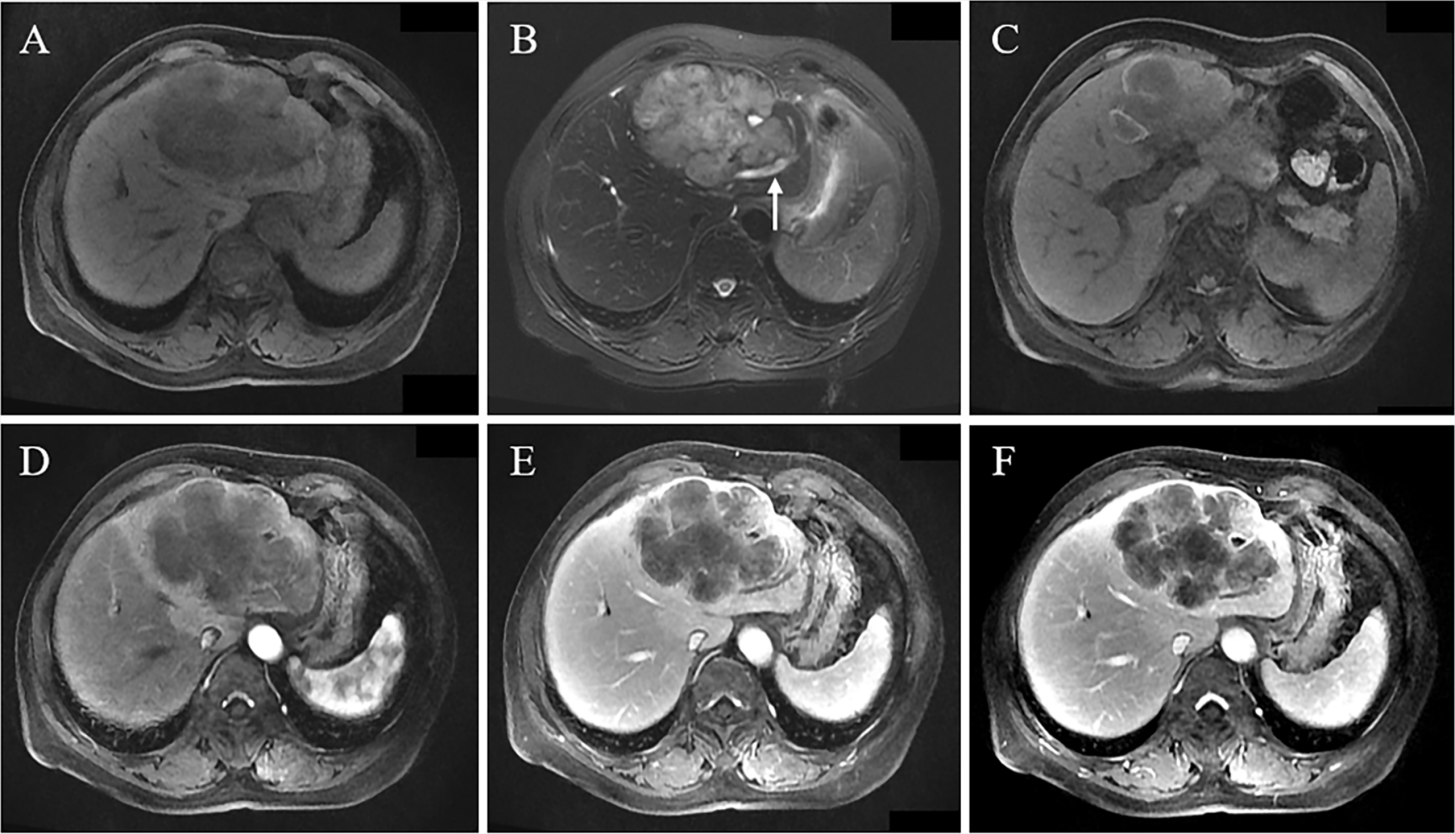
A 66-year-old female patient had abdominal pain. MRI revealed a huge lobulated mass in the left lobe, with hypointensity on T1WI (A), slight hyperintensity on T2WI, and local cholangiectasis (arrow) (B); local flake hyperintensity of tumor on T1WI reveals hemorrhage (C); Gd-DEPA enhanced MRI scan demonstrates peripheral enhancement of tumor in arterial phase, peritumoral abnormal perfusion (D), and multiple patchy enhanced regions of tumor observed in portal vein phase and delayed phase (E, F), indicating the progressive enhancement pattern.
